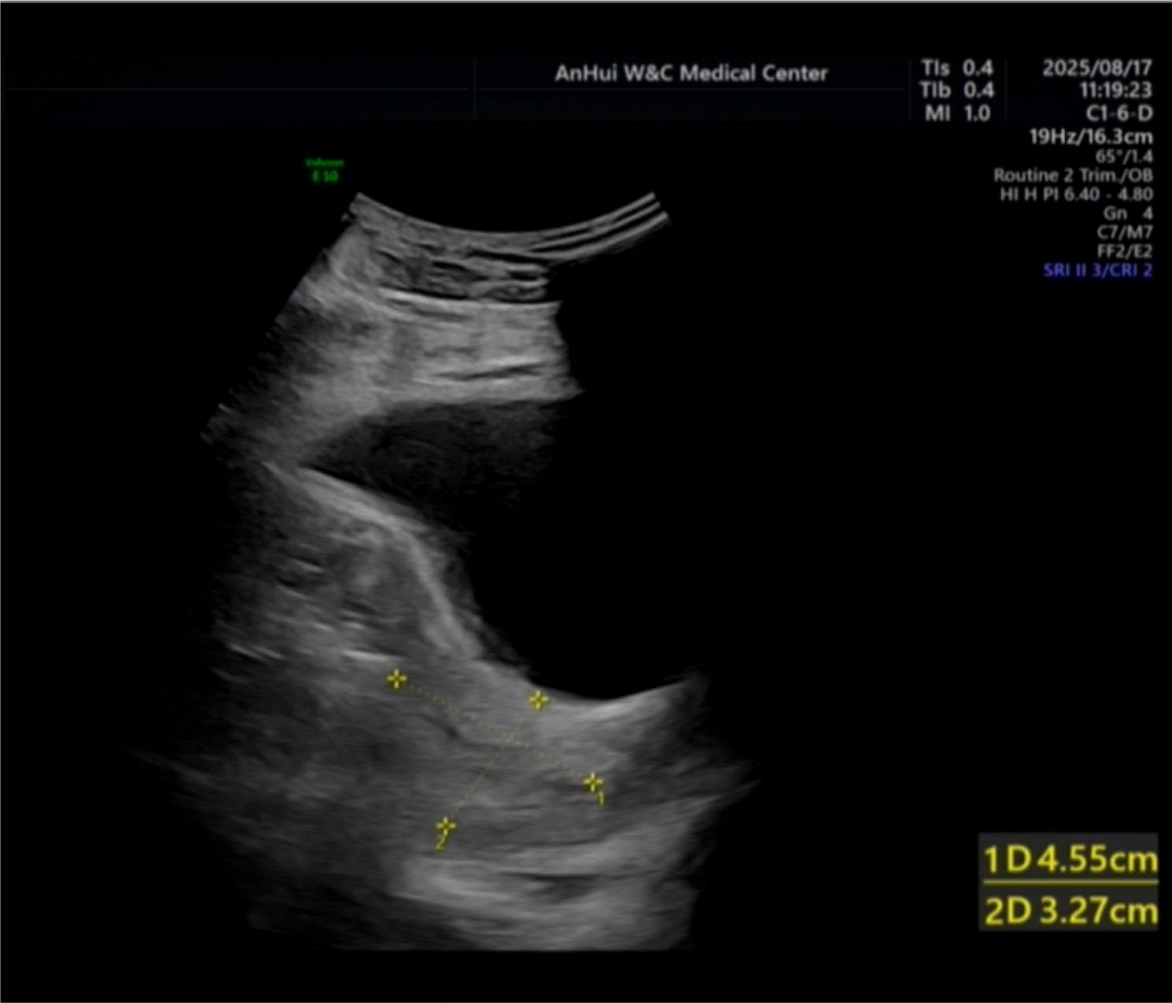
Postoperative transvaginal ultrasound image obtained one week after surgery.This sagittal view shows the lower uterine segment at the site of the repaired laceration and hysterotomy. The arrowheads point to the region of the healed scar, demonstrating a continuous and intact myometrial layer with no evidence of defect or hematoma. The endometrial stripe is visible and appears normal. These findings indicate satisfactory early healing following primary repair
